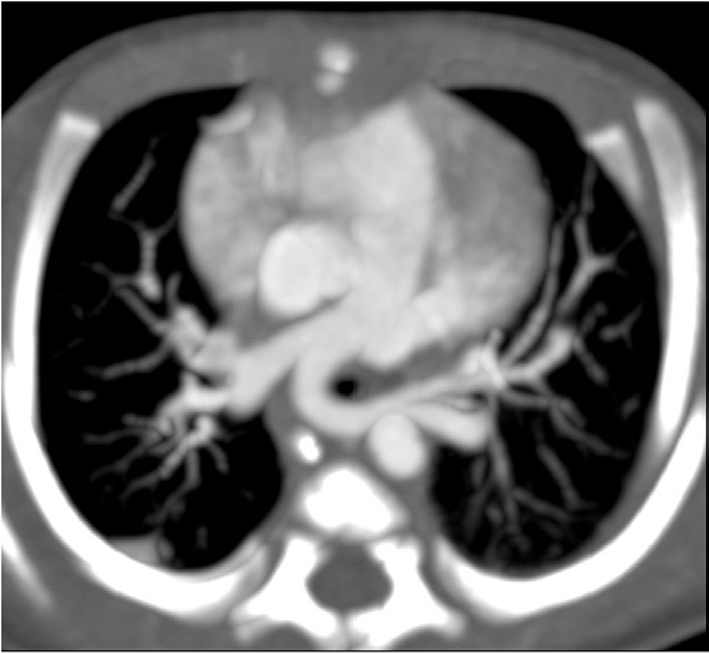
CT angiogram showing left pulmonary artery sling.
